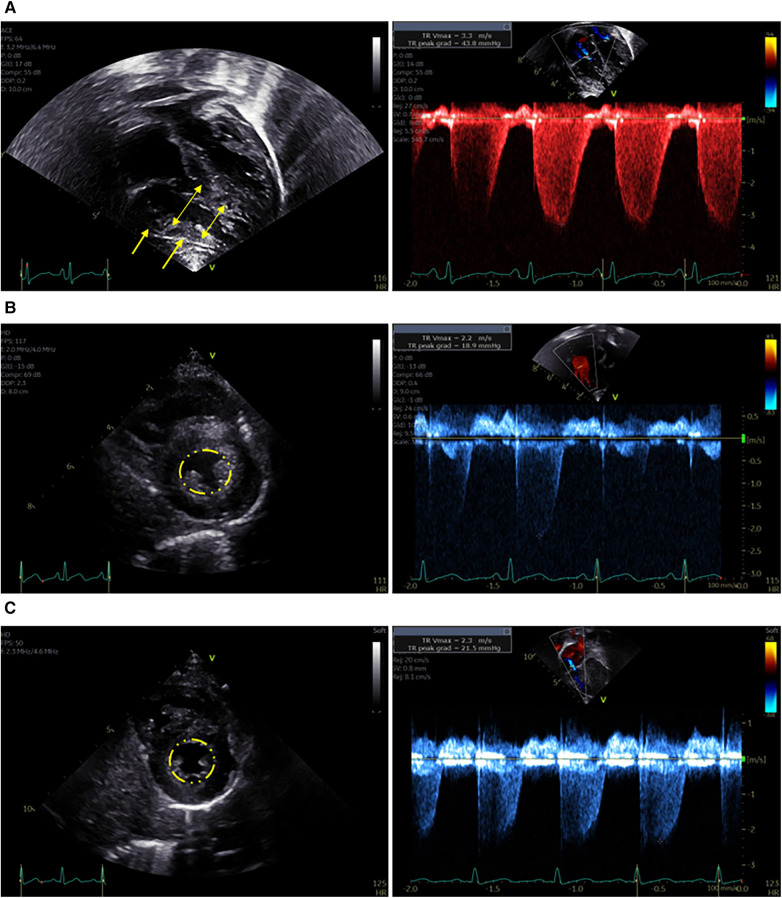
Representative images from echocardiograms obtained during the patient's course. (A) Immediately after cardiac arrest while on ECMO. Left panel: Apical 4-chamber view demonstrates right ventricular dilation (double headed arrows) and hypertrophy (single headed arrows). Right panel: Increased peak velocity of the tricuspid valve regurgitation jet indicates elevated right ventricular pressure. (B) Four weeks after cardiac arrest – on sildenafil. Left Panel: Parasternal short axis image demonstrates normal end-systolic septal position (indicated by circle), normal right ventricular size, and mild right ventricular hypertrophy. Right Panel: Normal peak velocity of the tricuspid valve regurgitation jet indicates normal right ventricular pressure. (C) Nine months after cardiac arrest – off sildenafil. Left Panel: Parasternal short axis image demonstrates normal end-systolic septal position (indicated by circle), normal right ventricular size, and no right ventricular hypertrophy. Right Panel: Normal peak velocity of the tricuspid valve regurgitation jet indicates normal right ventricular pressure.
